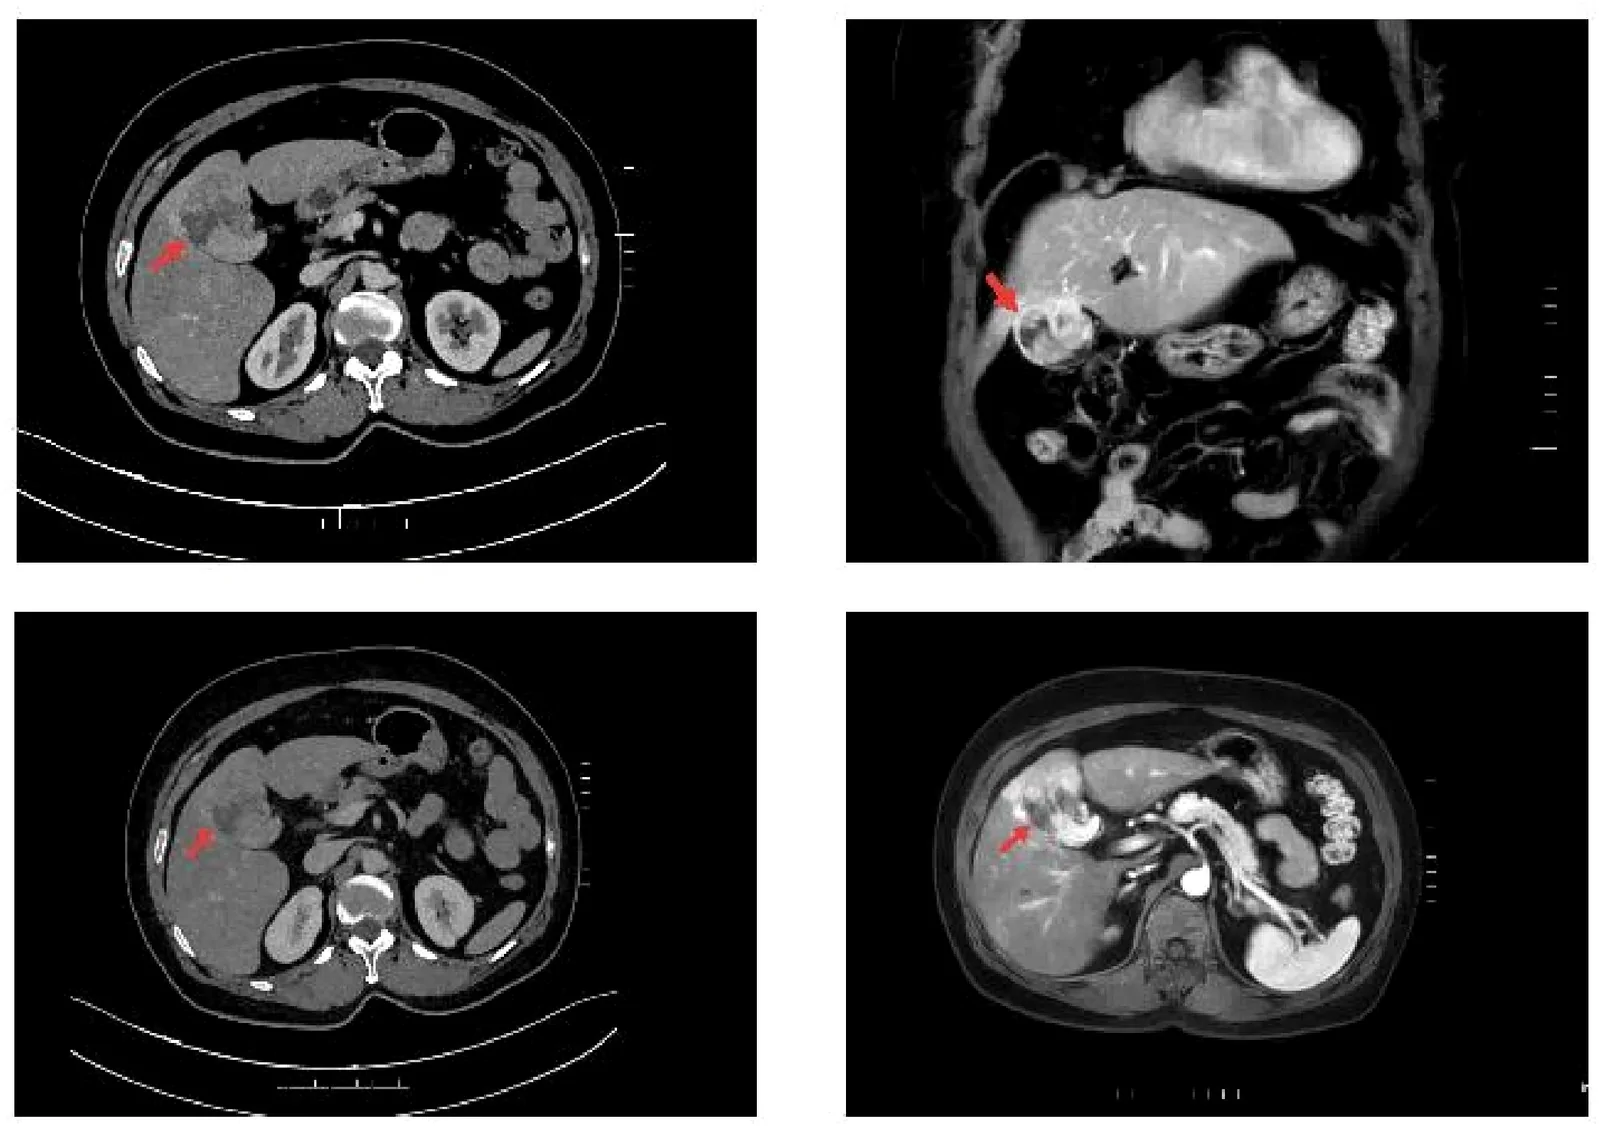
Imaging manifestations of gallbladder carcinoma. The two images on the left are contrast-enhanced CT of the whole abdomen, and the two on the right are contrast-enhanced MRI of the upper abdomen. The regions indicated by red arrows in the images represent the gallbladder carcinoma lesions. Photograph by author.
